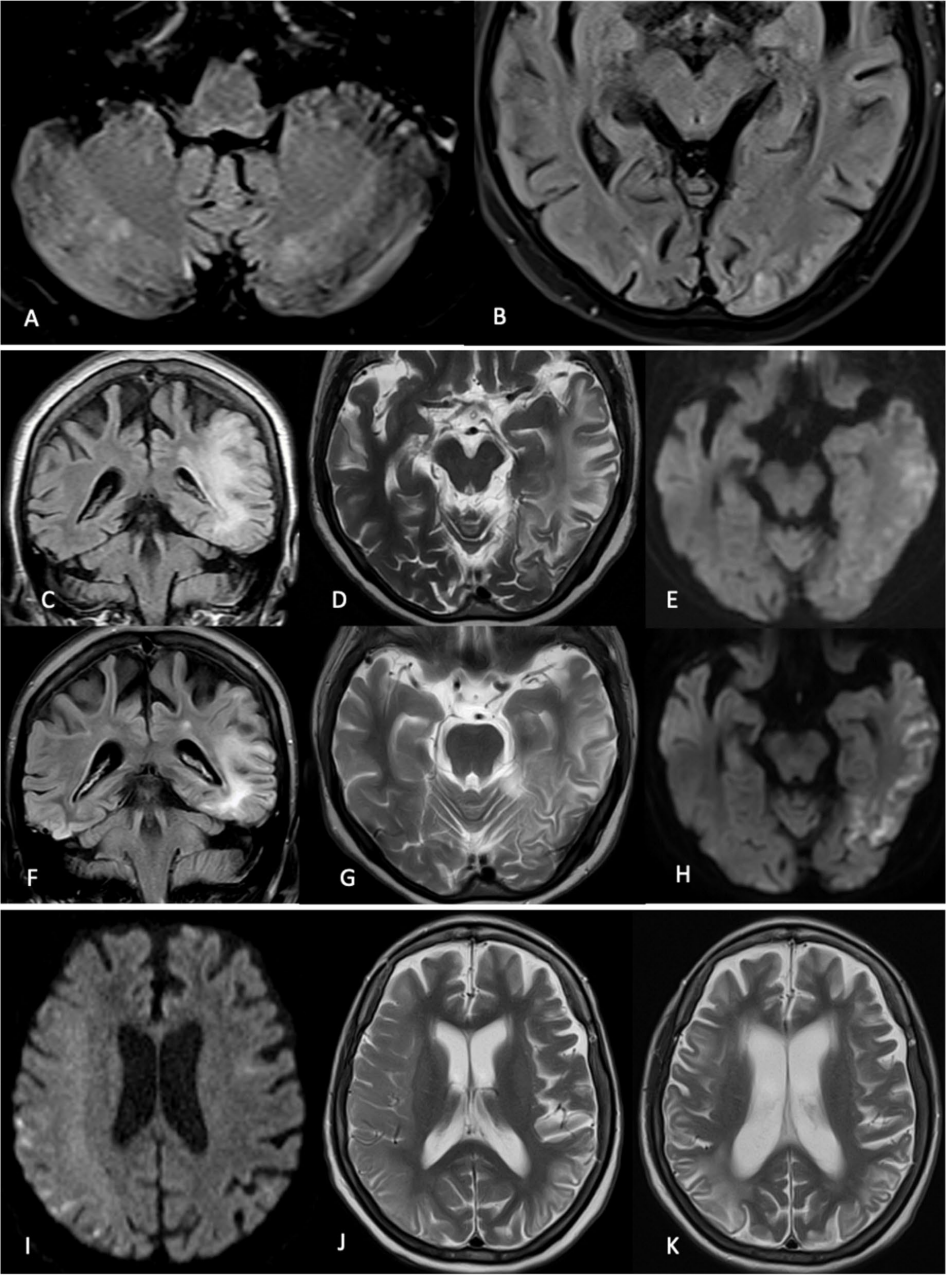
MRI Brain August 2017 FLAIR images showing (A) subtle bilateral cerebellar and (B) bilateral occipital cortical and subcortical white matter hyperintensities. These findings were thought to represent posterior reversible encephalopathy syndrome (PRES). MRI Brain in March 2019 (C, D, E) shows (C) left temporal and left parietal cortical and subcortical white matter FLAIR hyperintensities with vasogenic oedema, (D) left temporal and left occipital T2-weighted hyperintensities. E corresponding areas of patchy DWI hyperintensity. These new changes in the left cerebral hemisphere resemble stroke-like changes secondary to mitochondrial encephalopathy with stroke-like episodes (MELAS). Corresponding MRI Brain in June 2019 (F, G, H) shows temporal evolution of the changes observed in (C, D, E) with interval reduction of vasogenic oedema, stable DWI signal and interval development of gliotic changes. MRI Brain in March 2021 reveal right fronto-parieto-occipital cortical and subcortical white matter (I) DWI hyperintensity and (J) T2 hyperintensity with vasogenic oedema. MRI Brain in January 2022 reveal (K) interval development of gliotic changes in the right cerebral hemisphere. Note the pre-exisiting gliotic changes in the left cerebral hemisphere secondary to the stroke-like episode in March 2019. (A, B) Axial FLAIR; (C, F) Coronal FLAIR; (D, G, J, K) Axial T2-weighted; (E, H) Axial DWI
